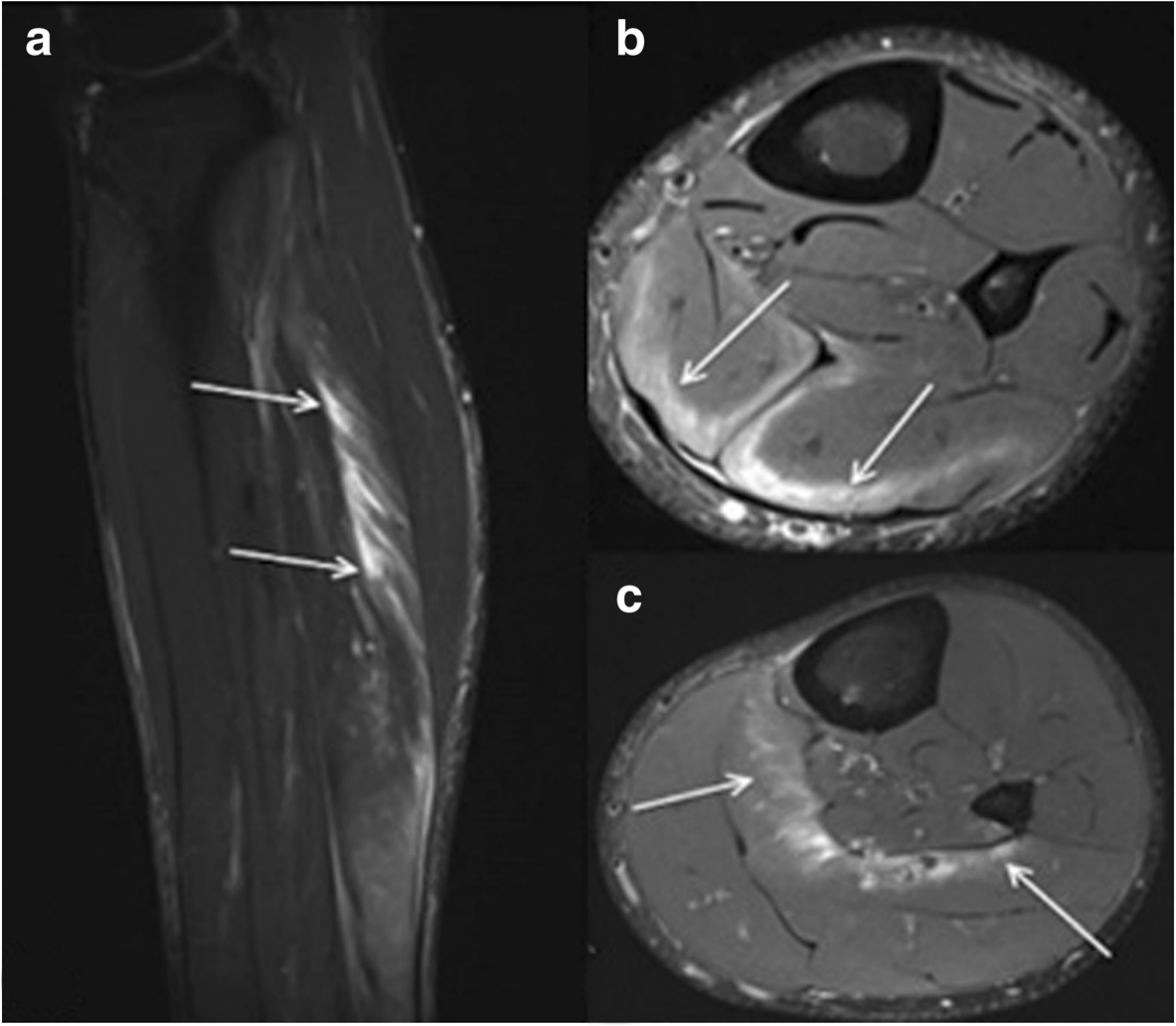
Sagittal STIR (a) and axial proton density-weighted MR images with fat saturation (b, c) showing extensive post-exercise edema within the soleus muscle in an Australian Rules football player. The edema is centered around the myotendinous junctions, demonstrating a geographically emarginated distribution (arrows). The patient played an entire game 2 days after this MRI. *Reprinted by permission from Springer: Springer Nature, Magnetic resonance imaging of the skeletal musculature, MRI of muscle injuries, Simon Dimmick, Christoph Rehnitz, Marc-André Weber, and James M. Linklater, 2014*
